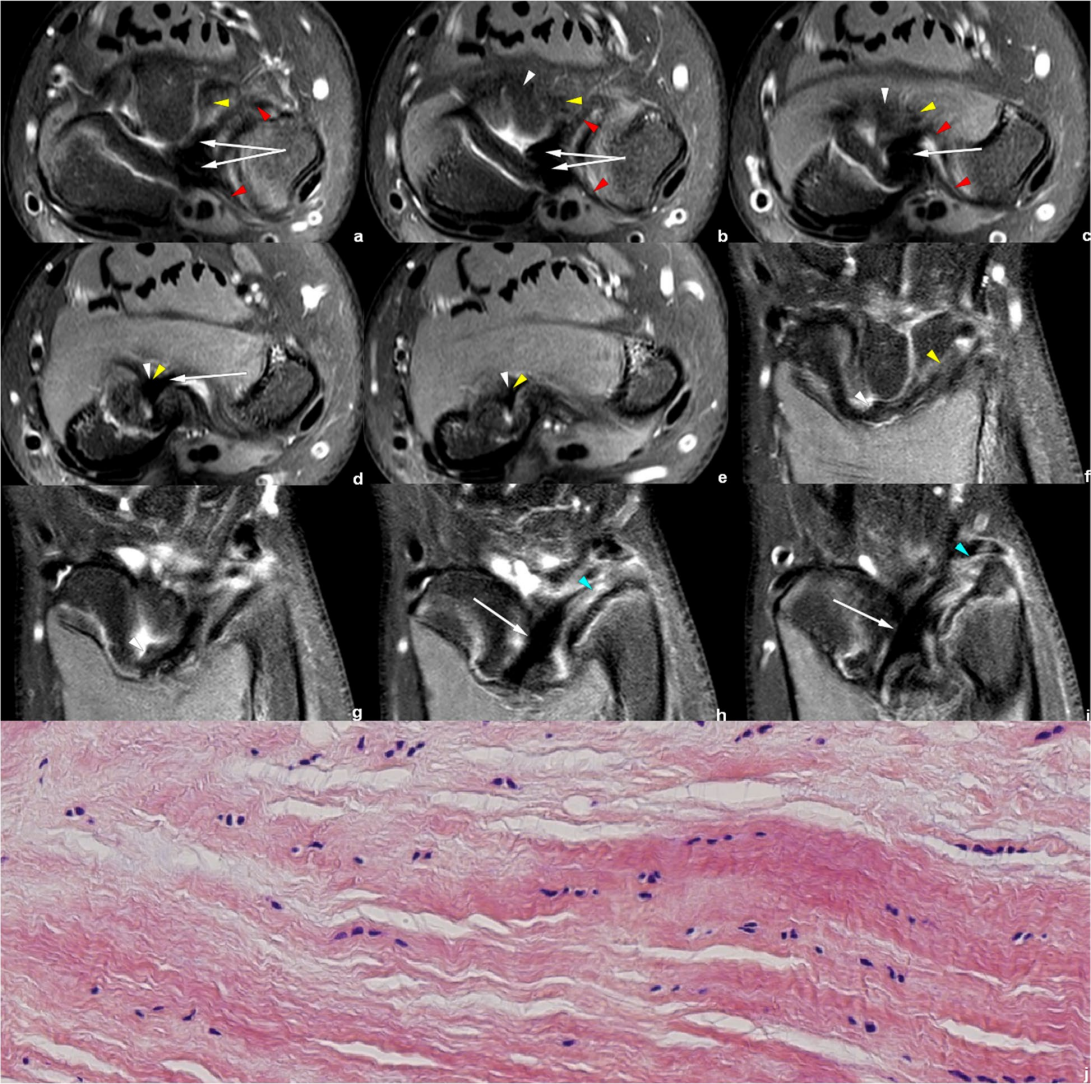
Left wrist MRI of the patient No. 0959. a-e Axial PDWI-FS images show the CD (white arrow), volar and distal radioulnar ligament (red arrowhead), VL (white arrowhead), and RTL (yellow arrowhead). f–i Coronal PDWI-FS images show the VL (white arrowhead), RTL (yellow arrowhead), and CD (white arrow). The blue arrowheads demonstrate the distal and proximal triangular ligaments attaching CD to the distal ulna. This patient underwent surgery to remove the “cord-like tissue connecting the distal radius to the volar aspect of proximal lunate.” j Pathological findings show disordered dense fibrous connective tissues and some fat
